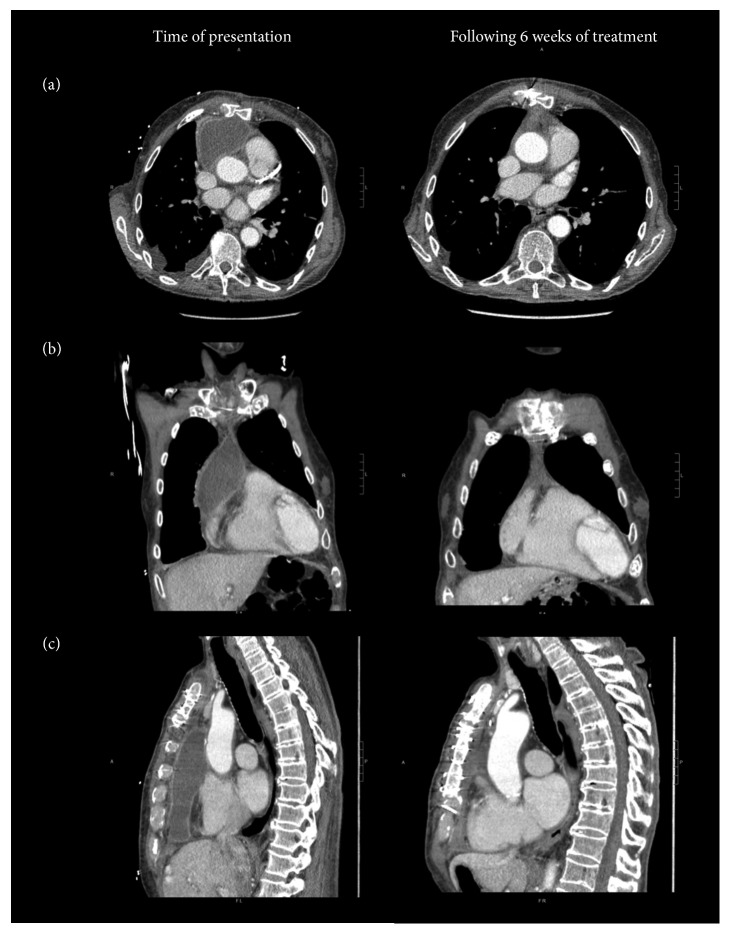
Computed tomography imaging with intravenous contrast showing nontyphoidal *Salmonella* mediastinal abscess. Panel (a) shows axial views, panel (b) shows coronal views, and panel (c) shows sagittal views at the time of presentation (left) and following 6 weeks of therapy with intravenous ceftriaxone (right).
